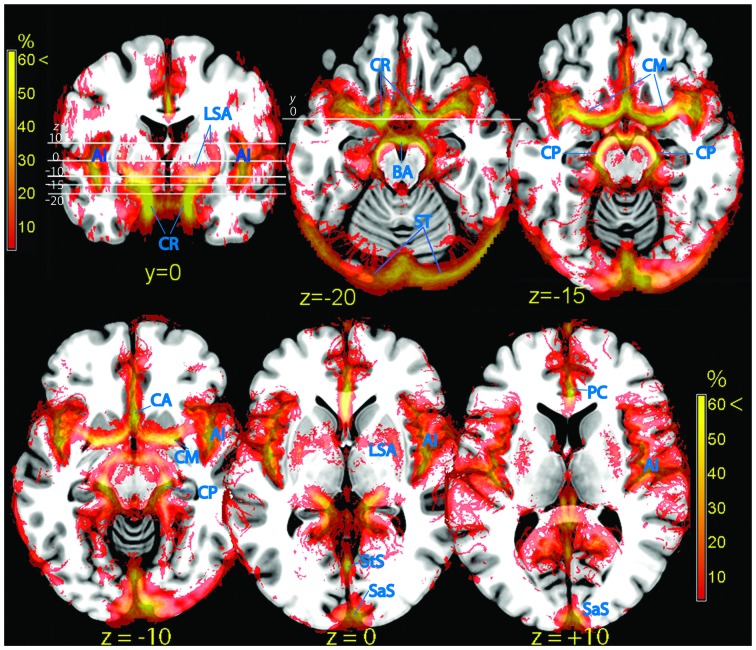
Vascular frequency maps in an axial slice located just superior to the circle of Willis (top row), and at the level of the insula (bottom row), overlaid on the ICBM high-resolution T1-weighted template, showing the major outlets of the internal carotis (anterior, middle, and posterior cerebral arteries). In the posterior part of the brain major sinuses are visible. The frontal section (top left) shows the internal carotis and the smaller vessels entering the ventral striatum from below. The high vascular frequency of the deep Silvian fissure, covering the insula, is also visible. Vessels (blue labels): LSA, lenticolo-striatal arteries; AI, insular arteries; CR, carotid artery; BA, basilar artery; ST, sinus transversus; CM, middle cerebral artery; CP, posterior cerebral artery; PC, pericallosal artery; StS, straight sinus; SaS, sagittalis sinus.
